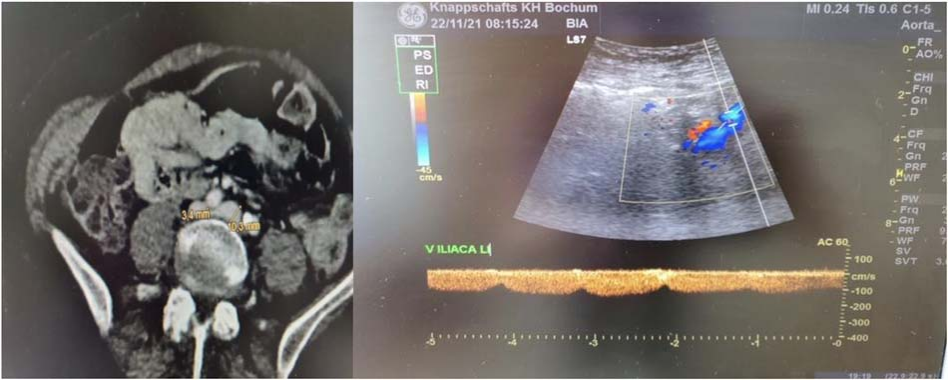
Radiological findings. Thrombosis of the left common/extern iliac vein as seen upon CT-scan and sonography.
